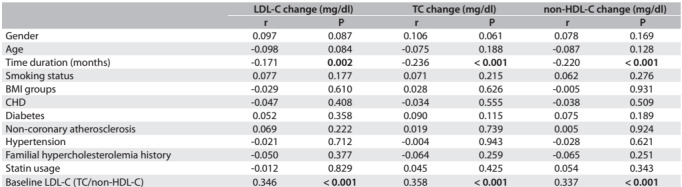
Magnetic resonance imaging of brain, four weeks after admission, showing: hyperintense image with T2 weighting (A); and enhancement after gadolinium-diethylenetriamine pentaacetic acid (Gd-DTPA) administration (B).
